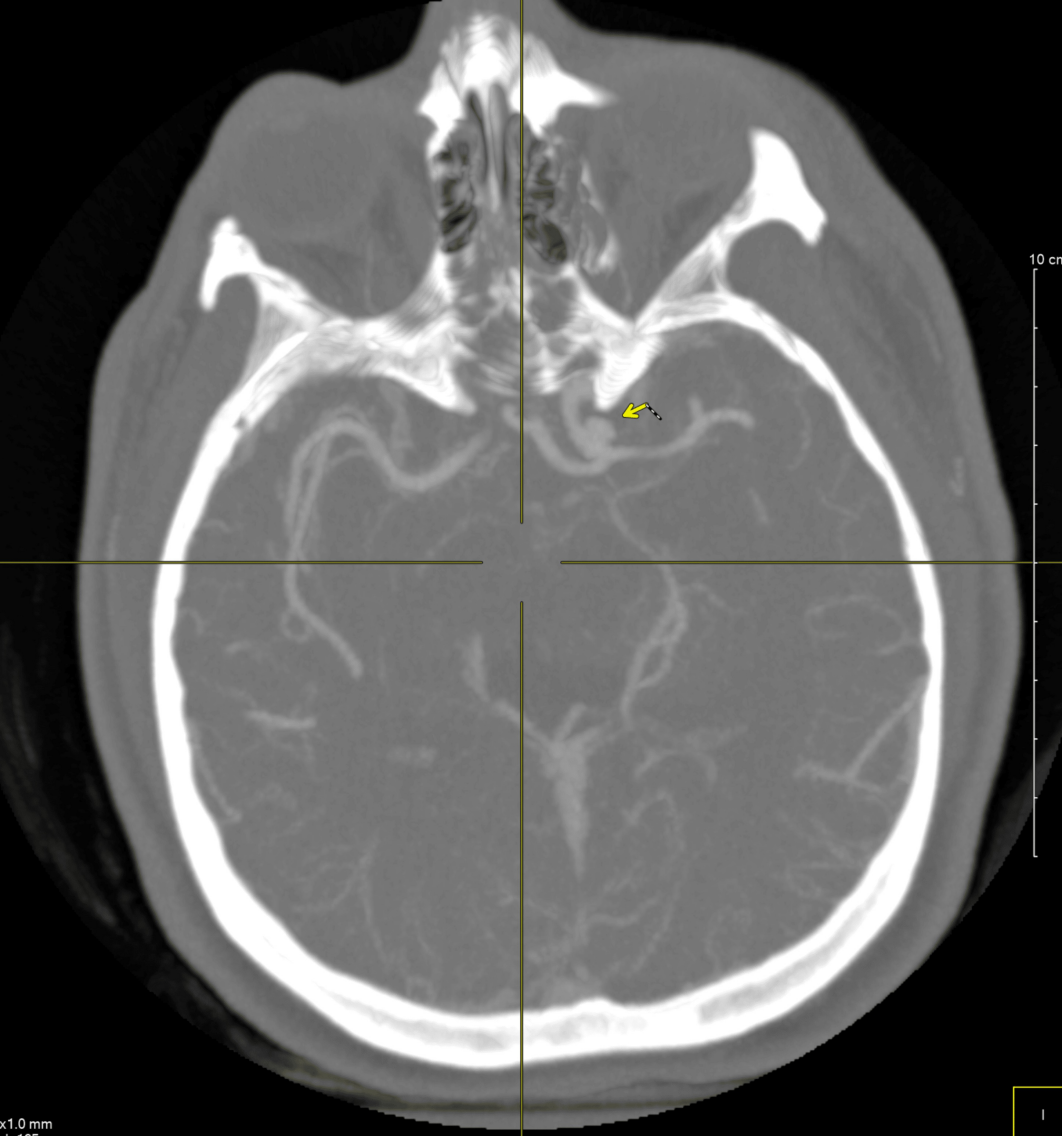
Axial CTA MIP reconstruction of the head demonstrating a left posterior communicating artery aneurysm (yellow arrow). MIP = maximum intensity projection; CTA = computed tomography angiography
